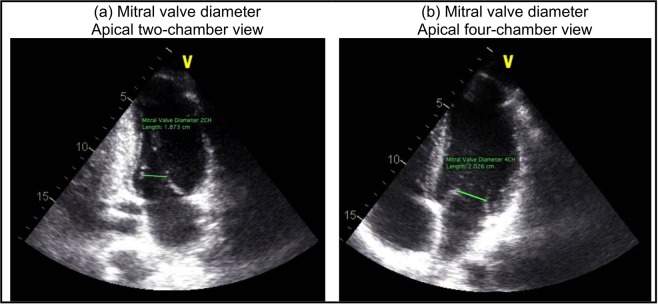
Mitral valve dimensions. (a) Mitral valve diameter (d_1_), measured in apical two-chamber view; (b) Mitral valve diameter (d_2_), measured in apical four-chamber view. Mitral valve is an ellipse and its area is quantified using A_MV_ =$$\frac{\pi \ast {d}_{1}\ast {d}_{2}}{4}$$.
